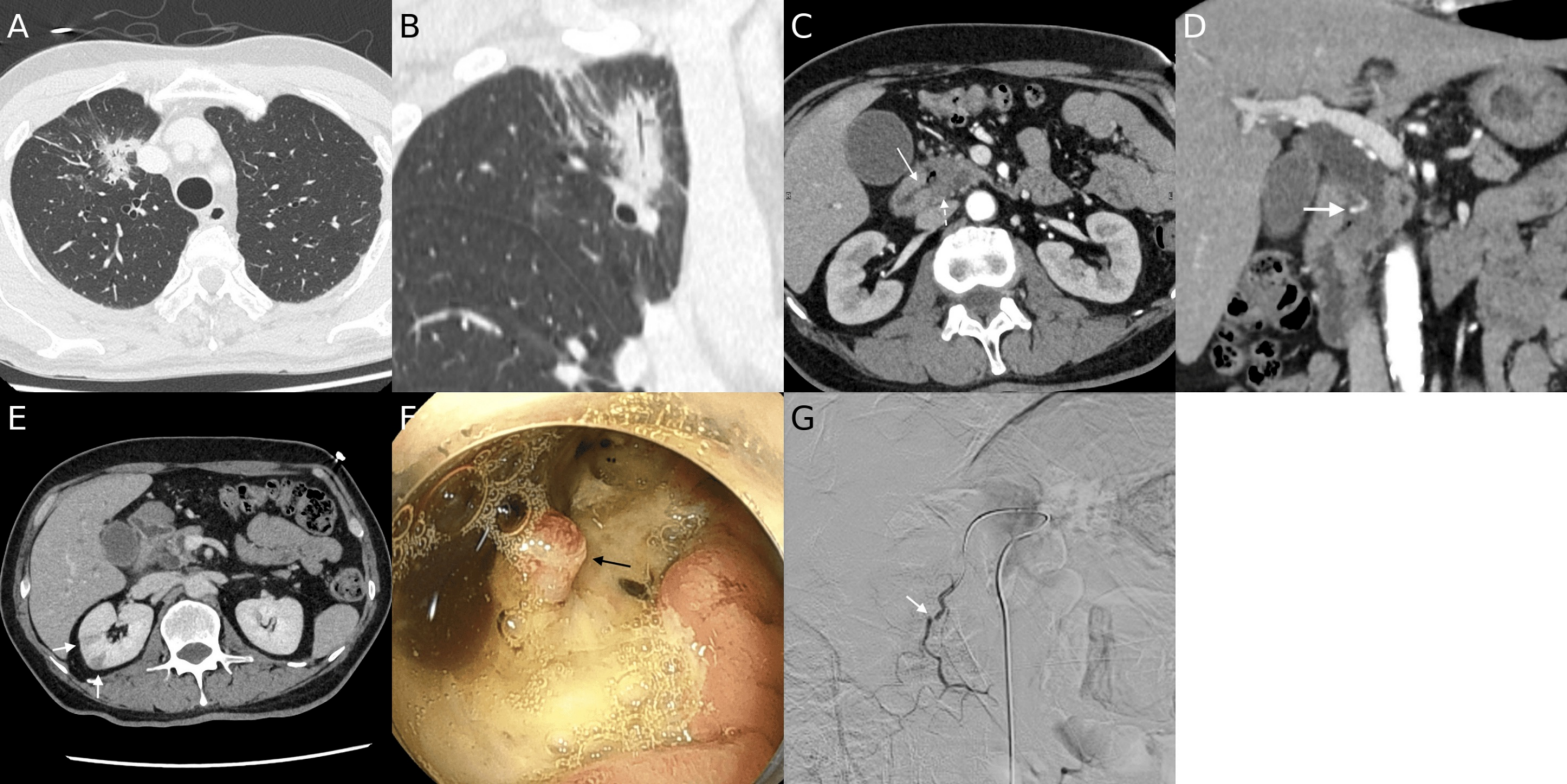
Initial contrast-enhanced computed tomography (CT) and angiographic findings at presentation (A, B) Right upper lobe pulmonary irregular consolidation, surrounded by ground-glass opacity and coarse spiculation, shown on axial (A) and coronal (B) images. (C, D) Hypovascular mass of the pancreatic head (dashed arrow). Axial image (C) demonstrates discontinuity of the duodenal wall (arrow), consistent with ulcerative invasion. Coronal image (D) shows biliary duct dilatation and a pseudoaneurysm of the posterior superior pancreaticoduodenal artery (PSPDA, arrow). (E) Wedge-shaped, hypoenhanced areas in the right kidney (arrow). (F) Upper gastroendoscopic image. A duodenal ulcer extending from the superior angle to the descending portion, with a 10-mm exposed artery at its center. No mass-forming lesion was identified. (G) Digital subtraction angiography (DSA) demonstrated disruption of the PSPDA at the location corresponding to the pseudoaneurysm previously identified on CT.
